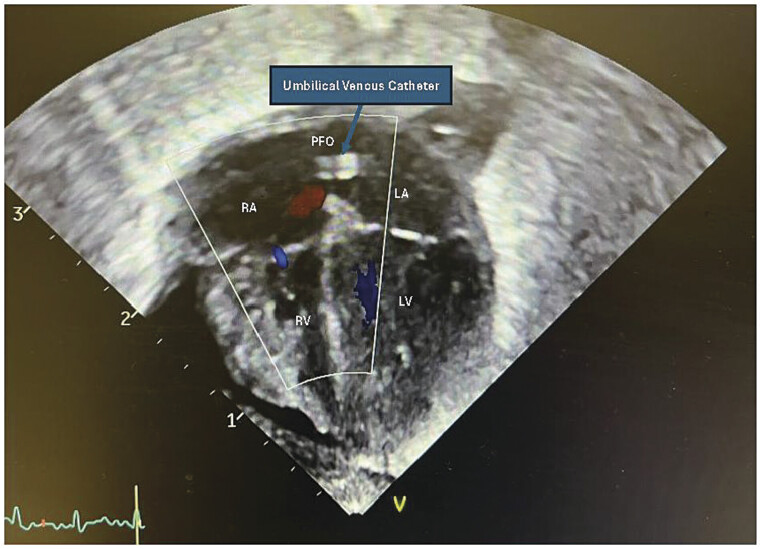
Echocardiography four-chamber view. Intracardiac migration of umbilical venous catheter (UVC) from inferior vena cava (IVC) into right atrium (RA) through patent foramen ovale (PFO) into left atrium (LA).
